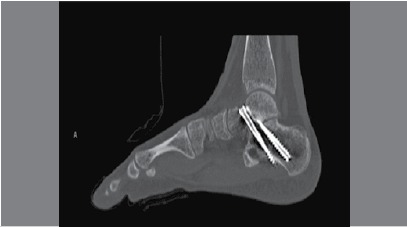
Postoperative sagittal CT scan cut showing nonunion at the subtalar joint arthrodesis site.
